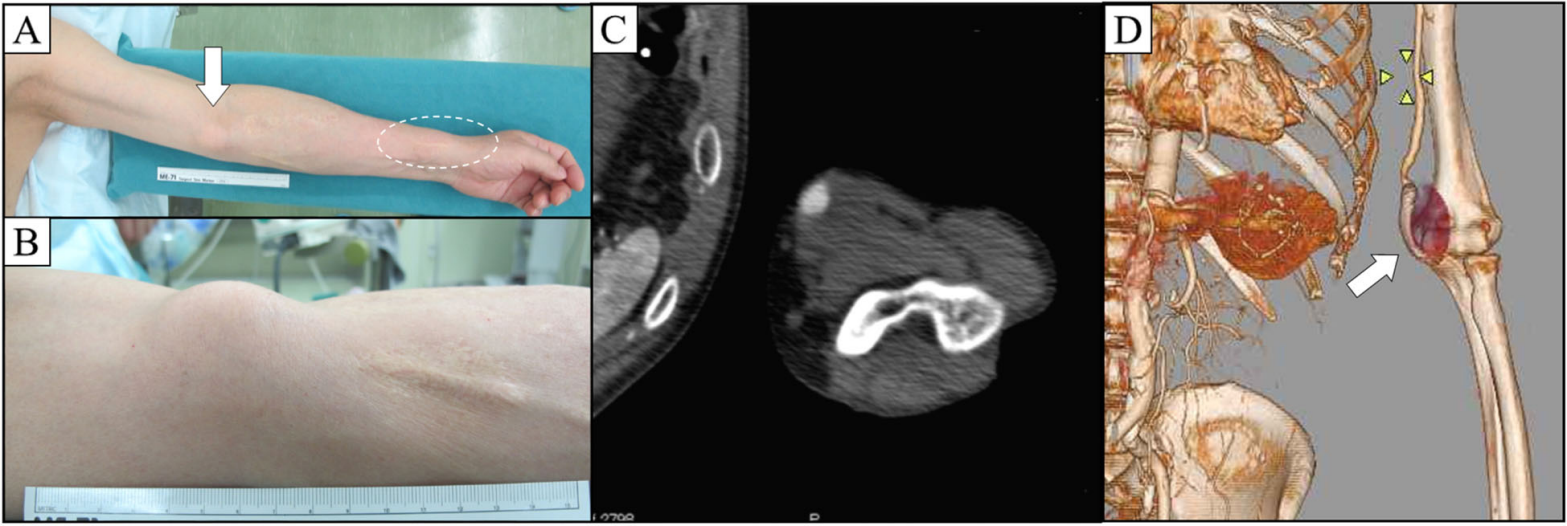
Preoperative imaging. a, b The left brachial mass was apart from AVF closure site (white dot circle). c, d Enhanced computed tomography showed a fusiform BAA with intramural thrombus and eccentrically patent lumen
